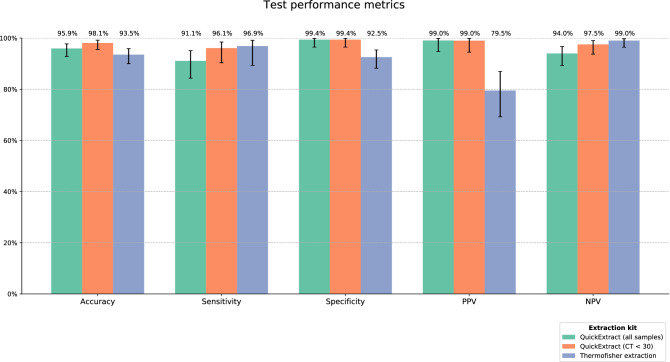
Performance results for the NGS approach in three different scenarios: (1) One-step RNA extraction using QuickExtract for all samples; (2) One-step RNA extraction using QuickExtract for samples with CT values lower than 30; (3) Regular ThermoFisher kit RNA extraction using MagMAX Viral/Pathogen II Nucleic Acid Isolation Kit. Error bars indicate 95% confidence intervals for the binomial proportions, calculated using Wilson score interval.
